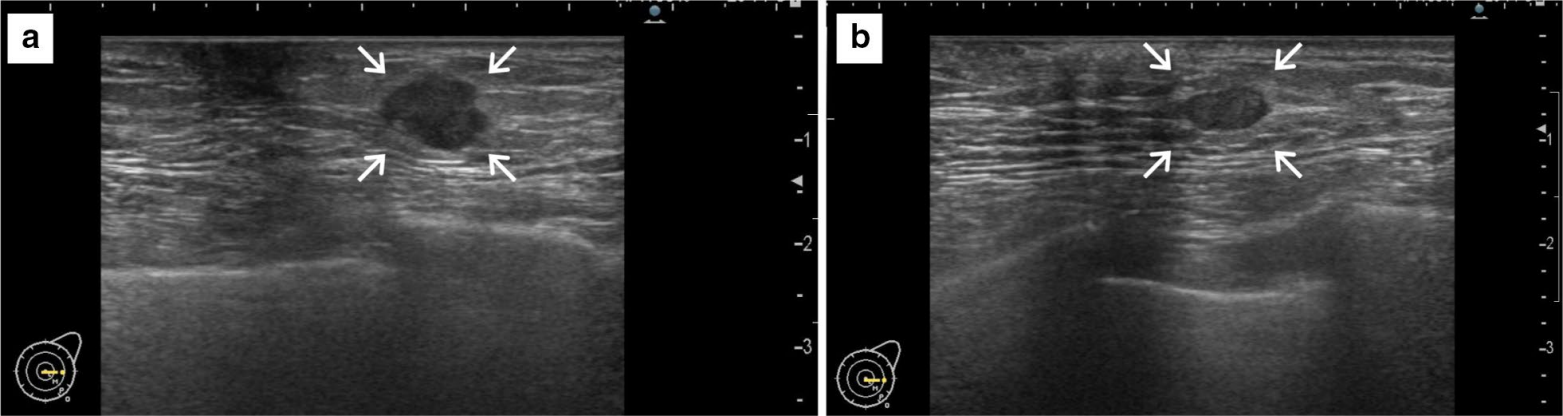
Ultrasonography findings. a On the patient’s first visit to our hospital, we observed a well-circumscribed, lobulated and hypoechoic lesion measuring 12 mm × 10 mm × 8 mm. b A week after the patient’s first visit, we observed a reduction in tumor size to 8 mm × 7 mm × 4.5 mm
